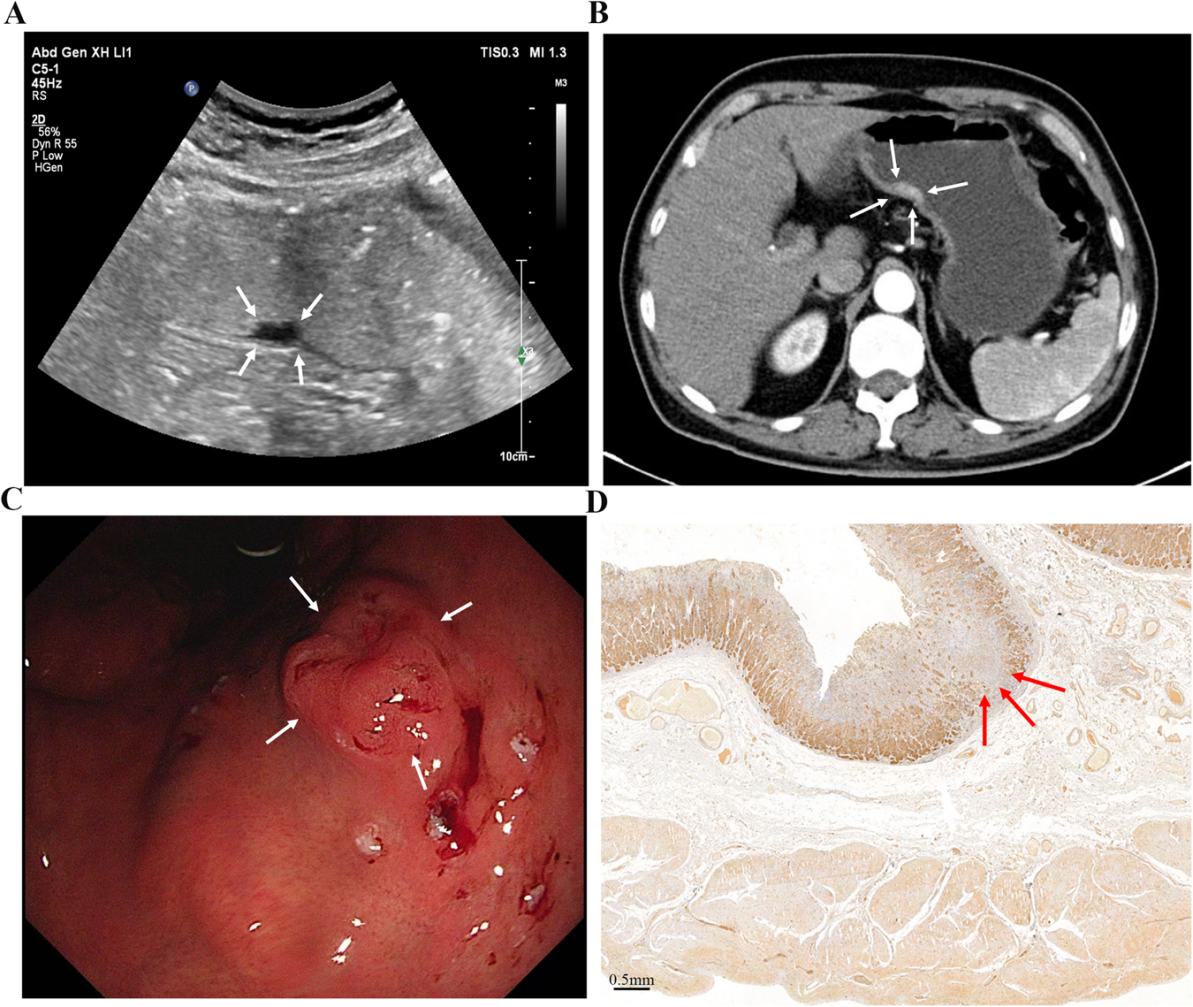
A An approx. 65-year-old man underwent gastric oral contrast ultrasonography, revealing a low echo in the mucosal layer characterized by an irregular shape on the lesser curvature of the gastric body (cT1). B The tumor showed local thickening and enhancement, indicating invasion of the muscularis propria layer of the posterior wall (cT2) in the lower part of the gastric body on enhanced computed tomography. C Electronic gastroscopy found a protrusion lesion on the lesser curvature of the gastric body, with intermediate ulcer formation. D The pathological result showed that the tumor invaded the submucosa (pT1) (scale bar: 0.5 mm)
